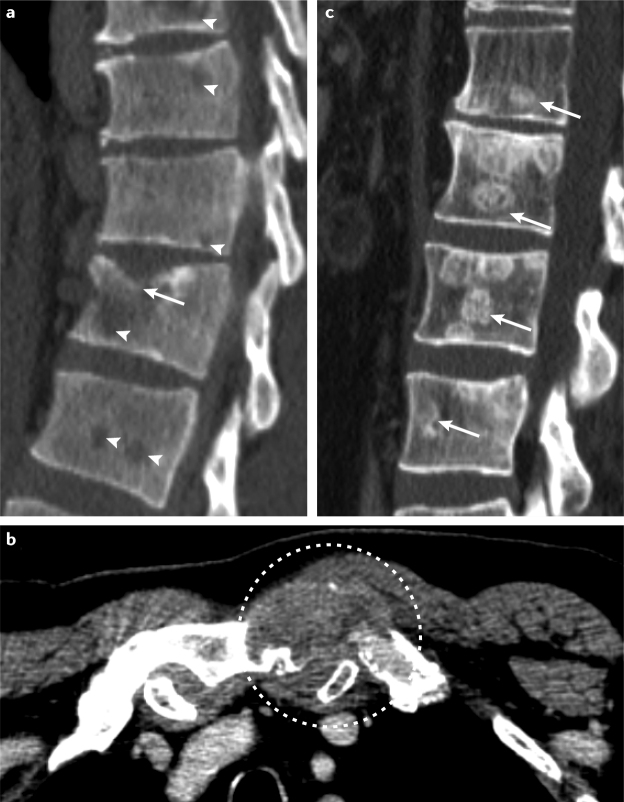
Radiographic pattern of RCC bone metastases. Bone metastases from renal cell carcinoma (RCC) can present in different patterns. a | Osteolytic bone metastases (arrowheads) that present with a symptomatic pathological fracture of the vertebral body (arrow) can be seen. b | Osteolytic bone metastases that present with an extraosseous soft-tissue portion in proximity to the sternoclavicular joint (dashed circle) can be seen. c | Bone metastases that result in bone formation (osteoblastic lesions) are rare (arrows).
